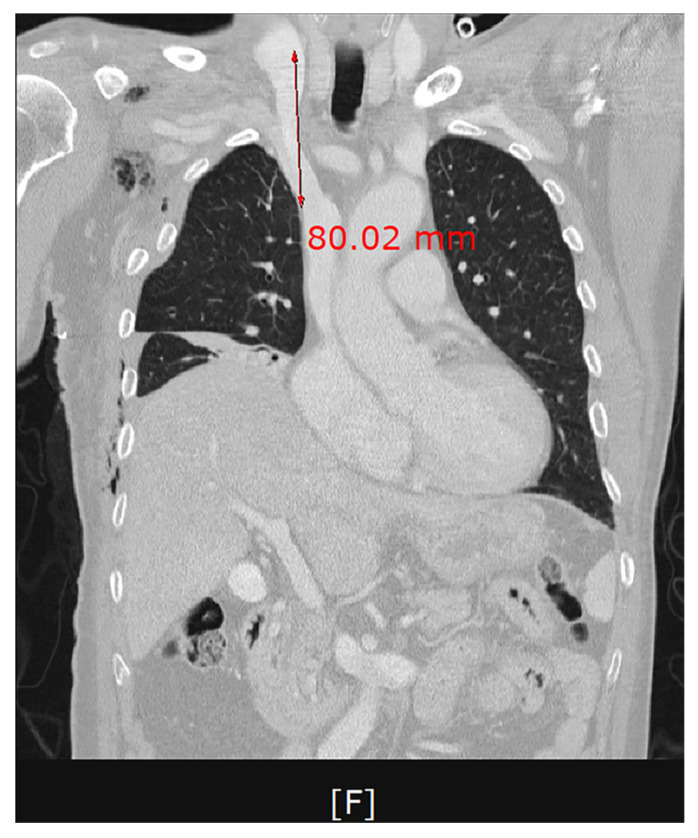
A frontal computed tomography scan of the chest. Approximately 80% of the length of a dilator corresponding to the distance between the site of puncture on the internal jugular vein and that on the superior vena cava.
